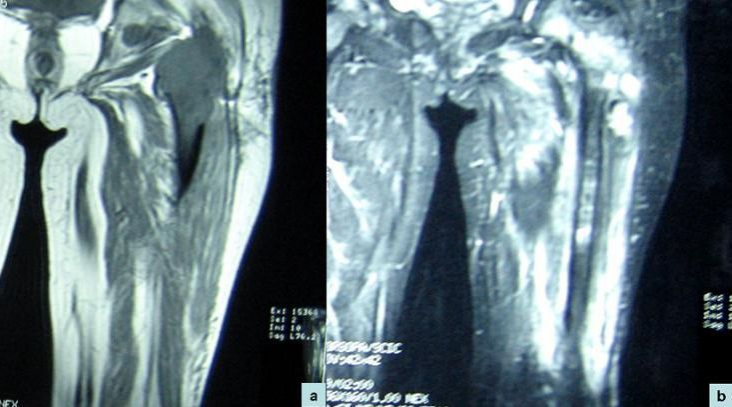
Magnetic resonance imaging shows evidence of lobulated soft tissue mass involving the upper end of left femur. (A) The mass was hypointense on T1-weighted imaging and (B) hyperintense on short inversion recovery imaging.
